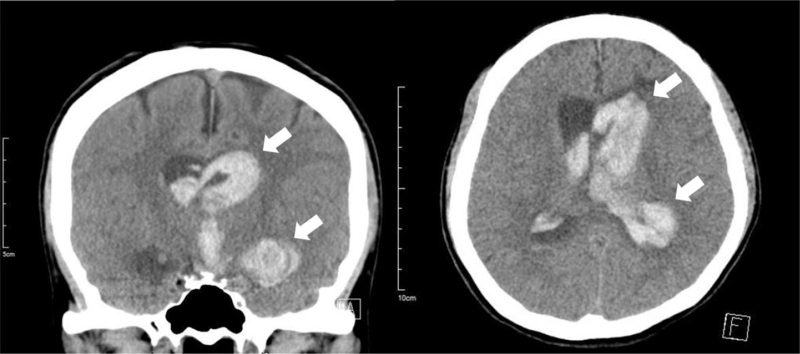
Urgent computed tomography scan of the brain without contrast revealed left caudate nucleus, temporal lobe intraventricular and intracerebral hemorrhage (white arrows) with hydrocephalus.
